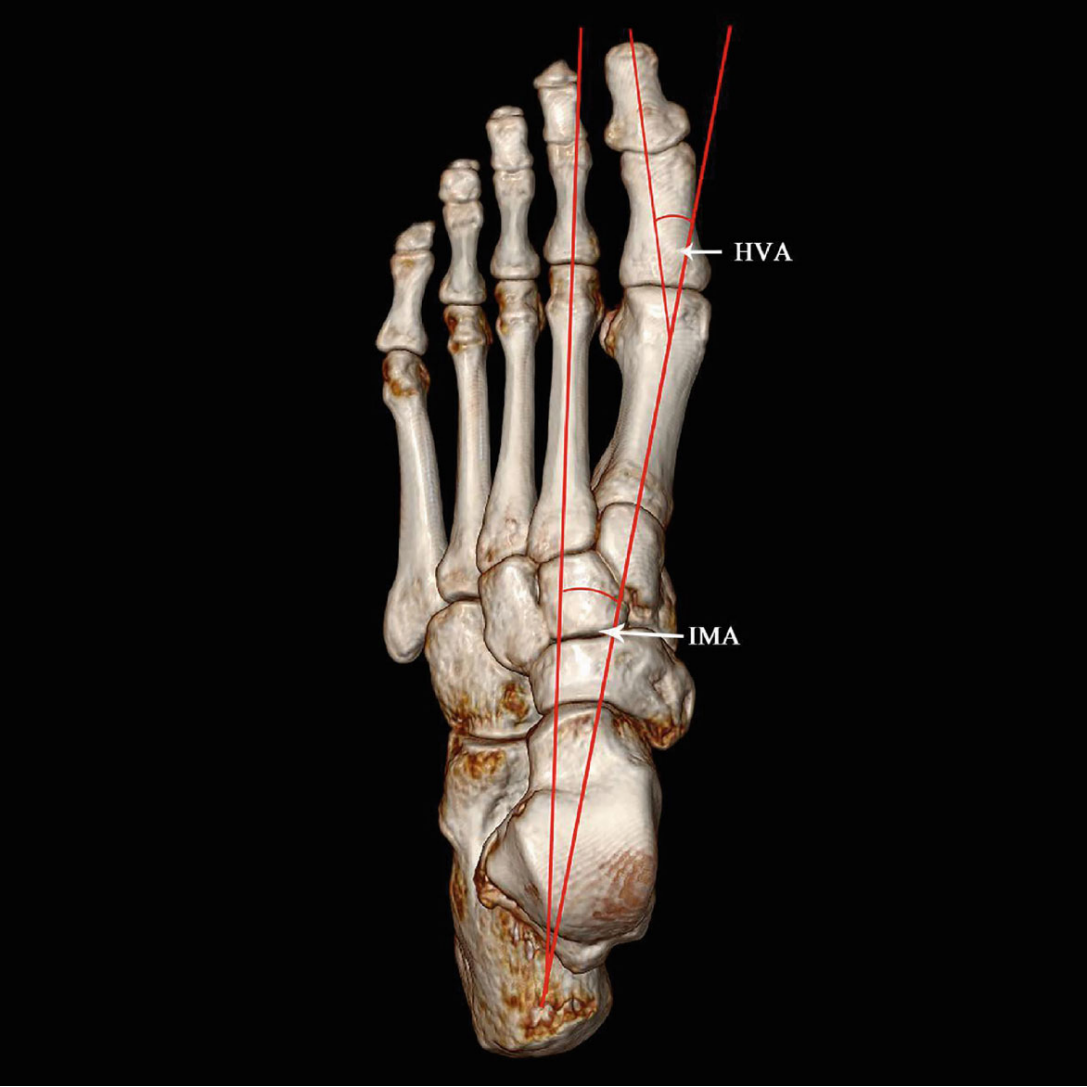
The angle to judge the severity of HV. On orthographic X-rays, the angle between the longitudinal axis of the first metatarsal bone and the longitudinal axis of the first phalangeal proximal phalangeal bone, normal < 16°. Intermetatarsal IMA: on the orthographic X-ray film, the angle between the longitudinal axis extension line of the first metatarsal bone and the second metatarsal bone, normal < 10°.
